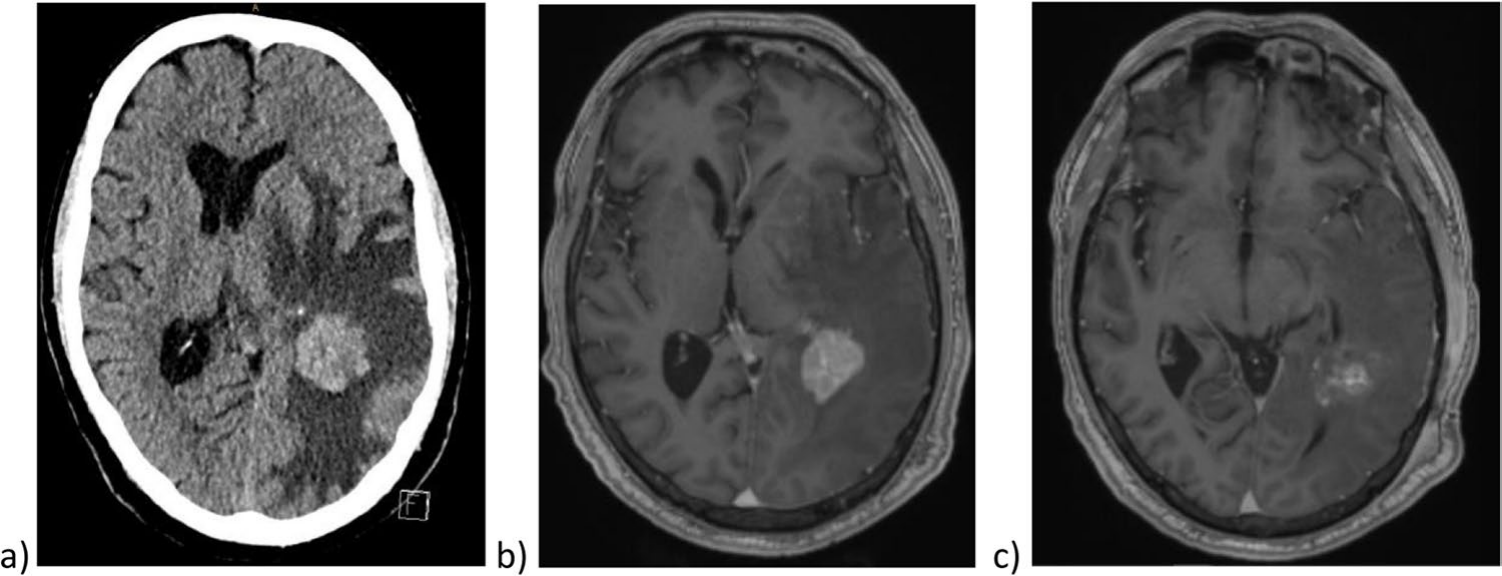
a Preoperative CT of the neurocranium, b preoperative brain MRI, and c MRI after surgery
